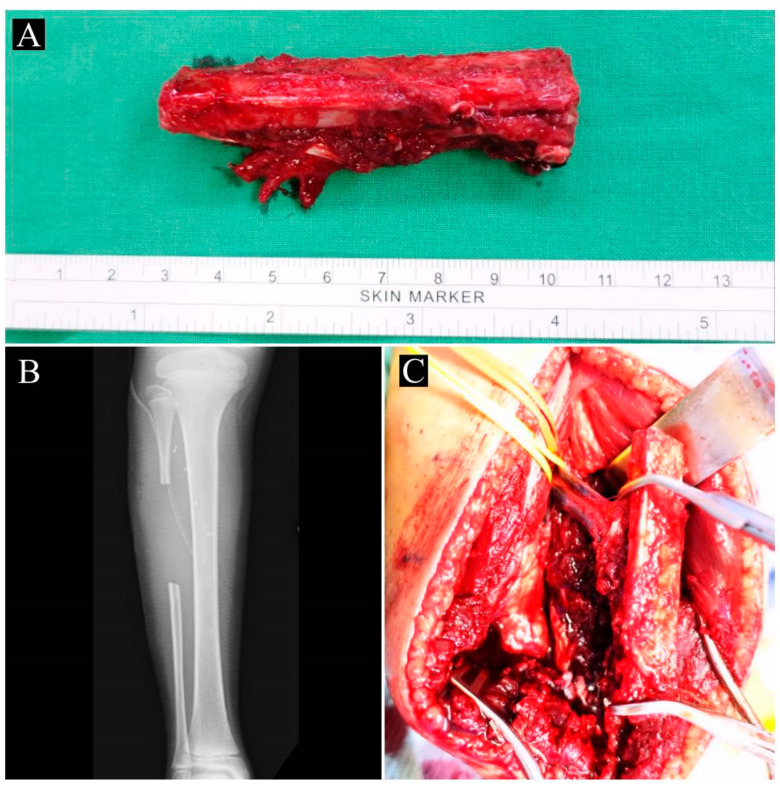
Harvesting the contralateral vascularized fibular graft: (A) harvested vascularized graft; (B) postoperative X-ray indicating well-preserved fibula beyond the mid-diaphysis region; (C) intraoperative image showing the donor peroneal artery and vein and fibular bone graft.
